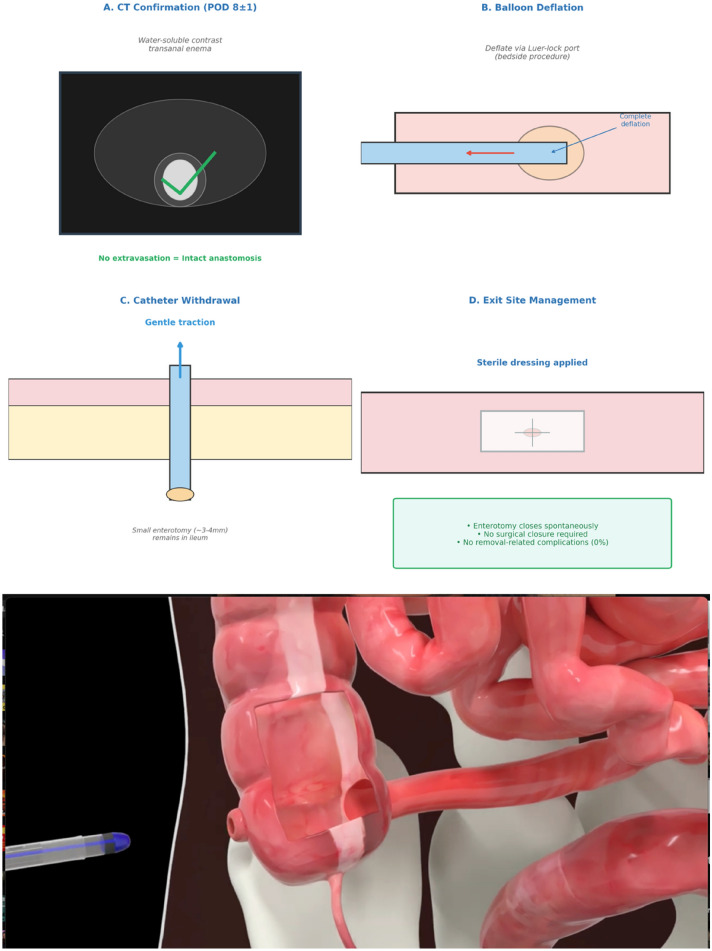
Device removal protocol. A CT with trans-anal water-soluble contrast confirming anastomotic integrity at postoperative day 8. B Balloon deflation via Luer-lock port. C Catheter withdrawal. D Exit site with enterotomy (allowed to close spontaneously) and sterile dressing application
